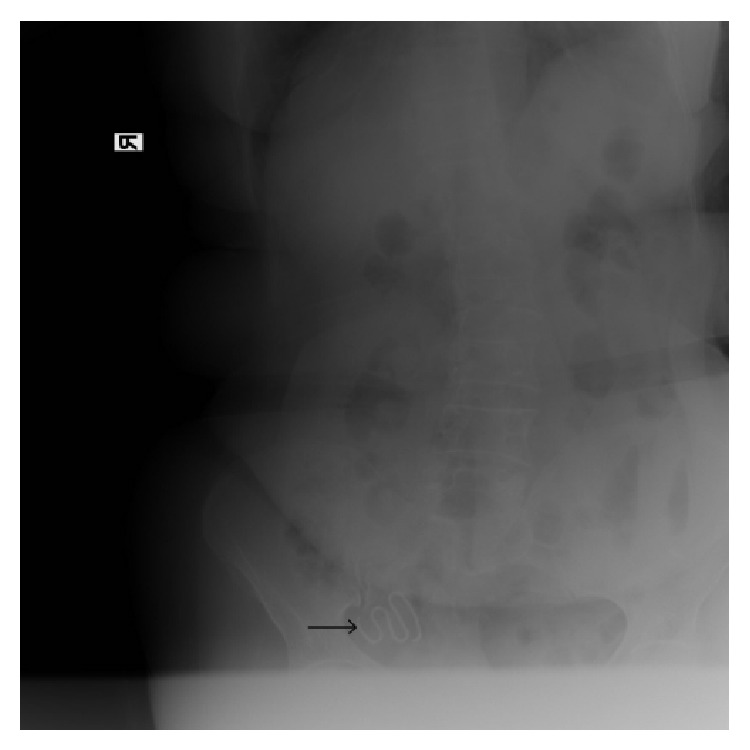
Pelvic X-ray reveals a Lippes loop IUD in the pelvis.
